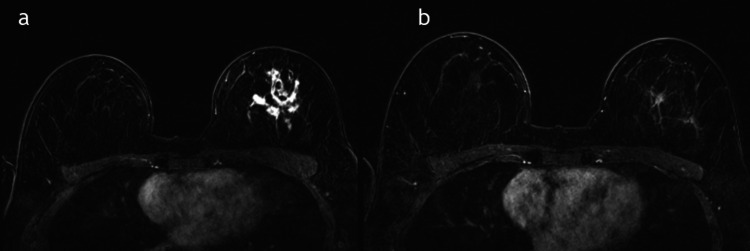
MRI pre and post-NAC. A 45-year-old female patient with left-sided invasive ductal carcinoma; (a) postcontrast MRI before the start of NAC shows multifocal enhancing foci in the left breast, and (b) postcontrast MRI after NAC shows residual disease. MRI: magnetic resonance imaging; NAC: neoadjuvant chemotherapy
